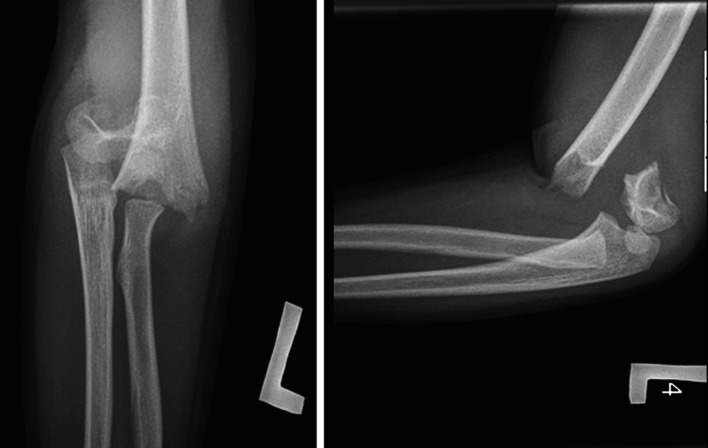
X-ray of third type of supracondylar humerus fracture
